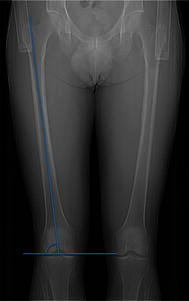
Measuring of varus or valgus angles of the distal femur using the tomograms. Varus or valgus angles of the distal femur were measured between the long axis of the femur and the joint surface of the distal femur. As the spiral computed tomography (CT) was obtained only in the lying position, a proper evaluation of mechanical and anatomical axes, which should be performed in an erect position for ambulating individuals, could not be performed
